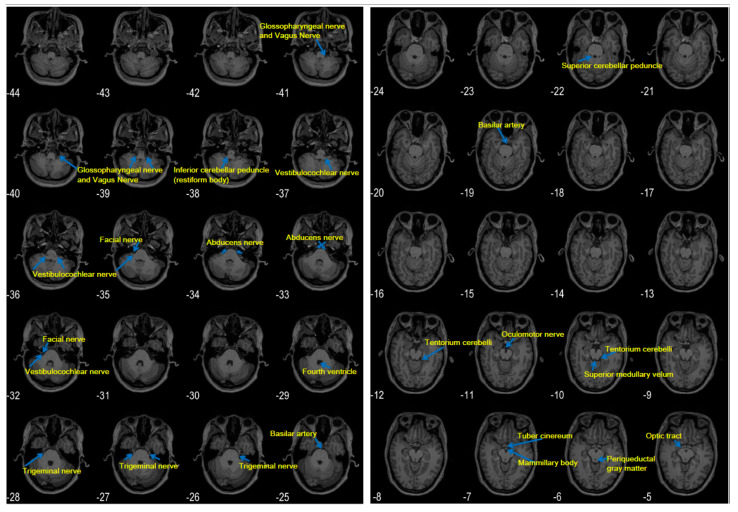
Cranial nerves in high-resolution magnetic resonance (MR) images. Images were produced by using the statistical parametric mapping (SPM) toolbox. High spatial-resolution T1 images (0.5 mm × 0.5 mm in the axial plane, 1 mm slice thickness) were collected with a 3T magnetic resonance imaging (MRI) scanner. Numbers indicate locations in the z-axis. Note the different structural features between (z=) “−37,” showing the root of the vestibulocochlear nerve, and (z=) “−39,” showing the roots of the glossopharyngeal/vagus nerves. In these high-resolution images, the root of the glossopharyngeal and vagus nerves cannot be visually separated. Structures are indicated on the basis of References [34,35].
